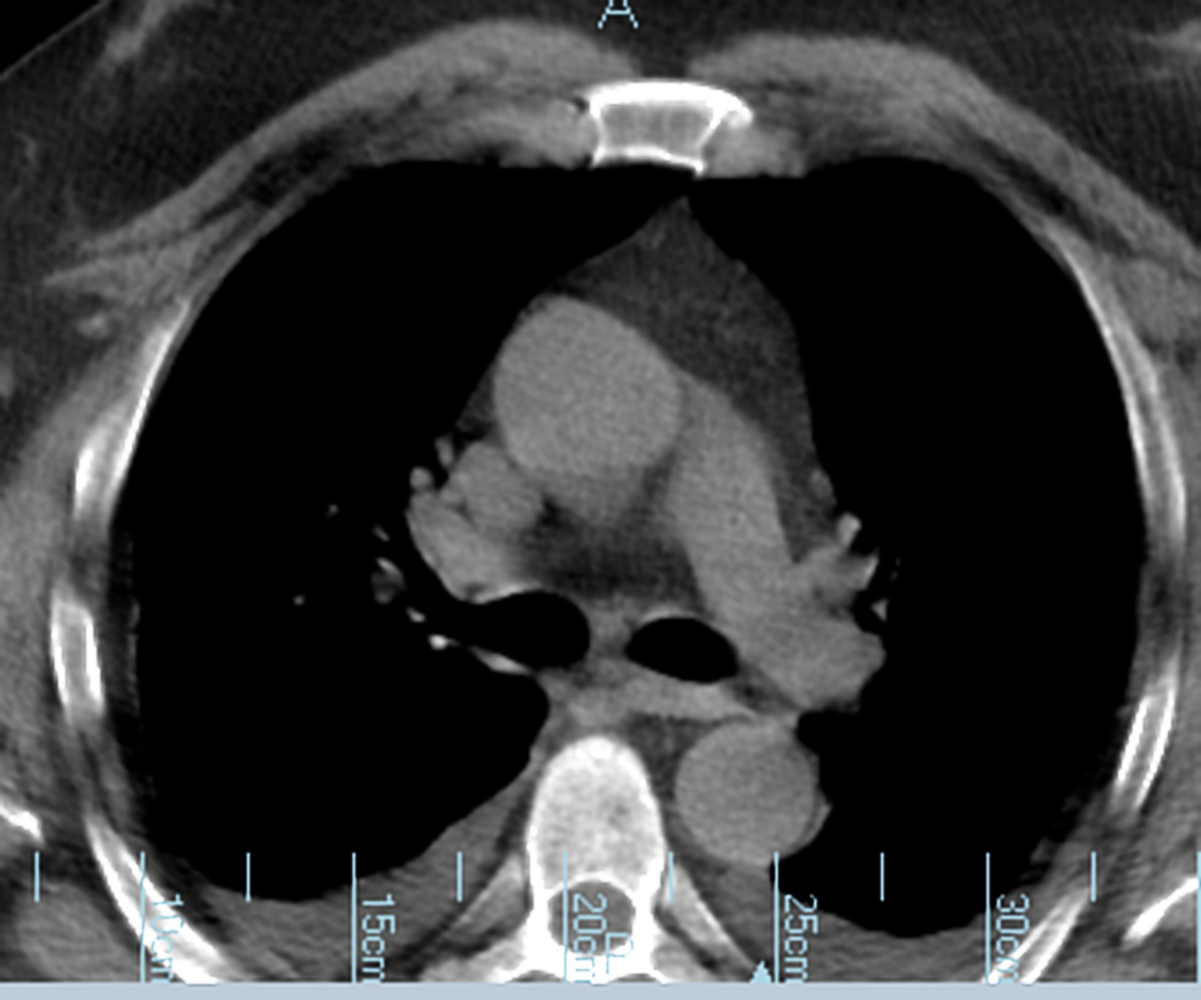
Noncontrast chest computed tomography scan, showing no abnormalities in the chest.
